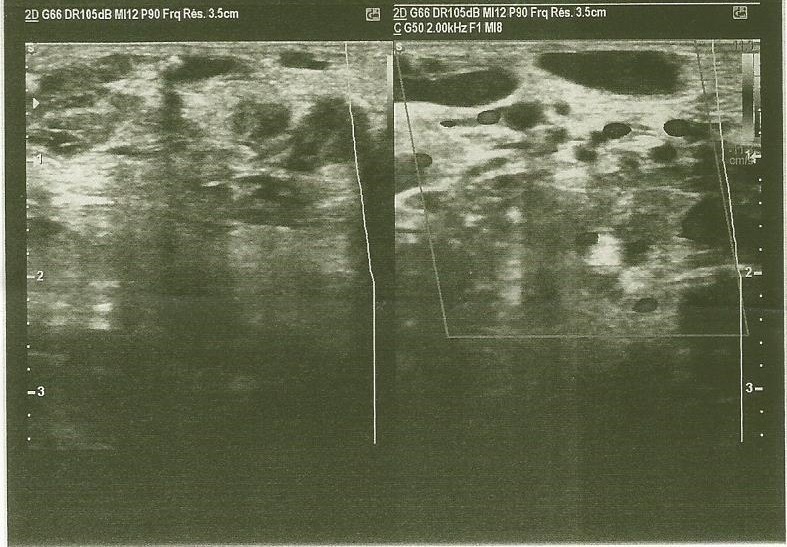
Echographie doppler de la MAV: fistules artério-veineuses (FAV) multiples
